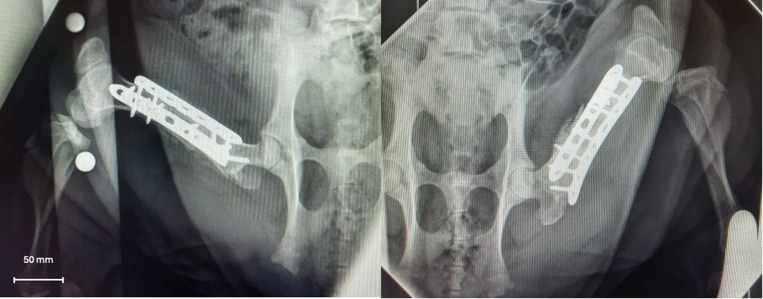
Postoperative radiographic controls: control of the correct position of the graft on each side (day 15).
